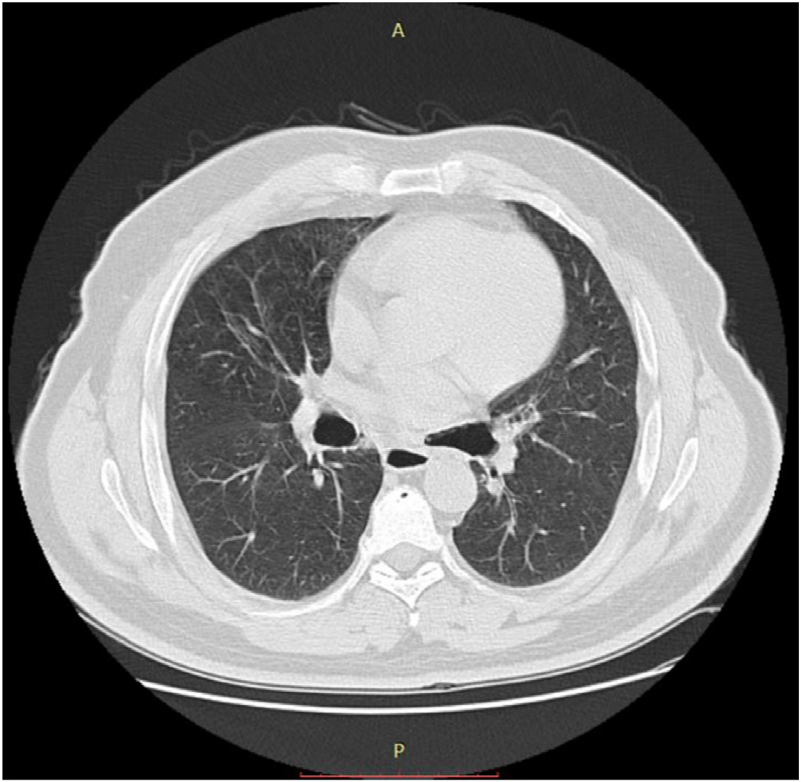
Thoracic CT scan: axial sections through the parenchymal window showing dilatation of the right and left main bronchi.
